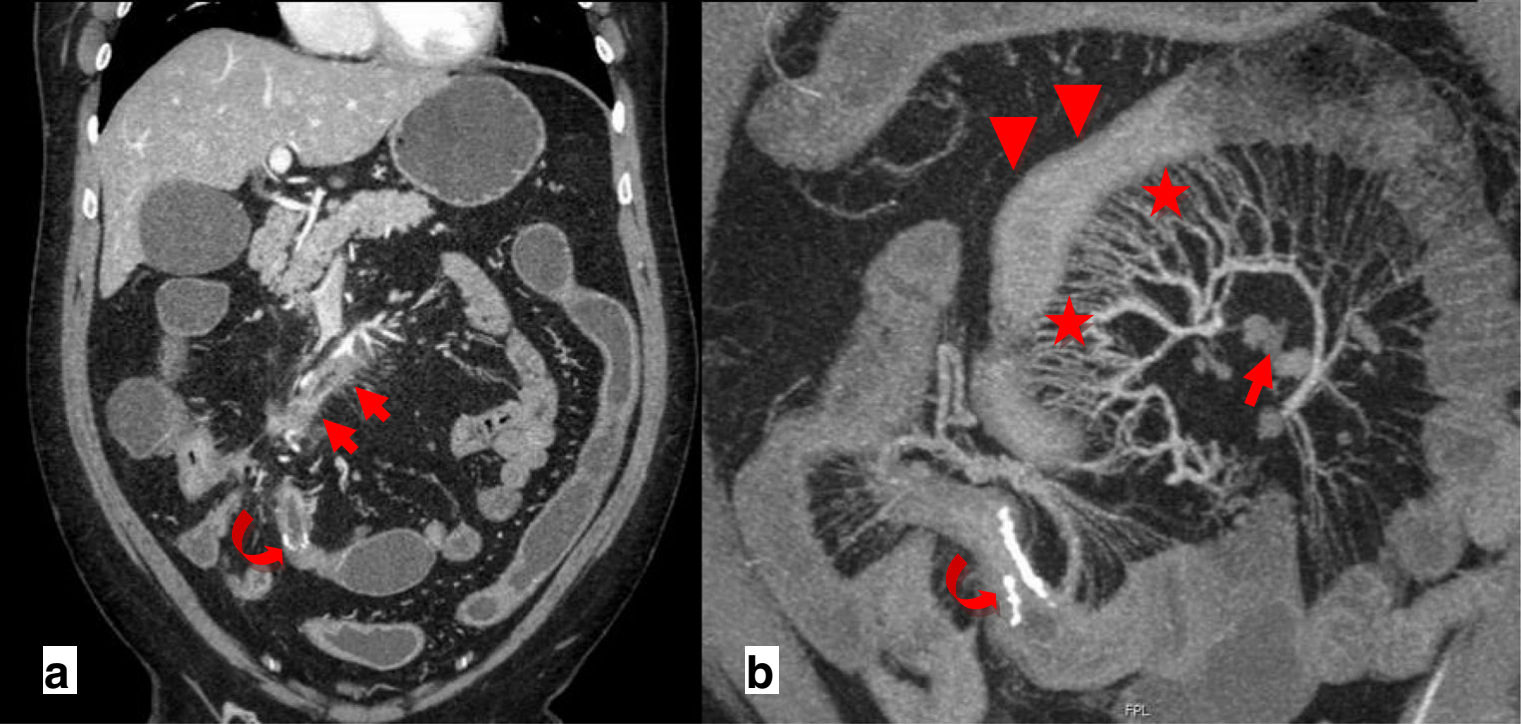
a, b CT enterography of a patient with Crohn’s disease who had previously undergone segmental enterectomy. Note the surgical material at the ileal-ileal anastomosis (*curved arrows in a, b*). There is a fistulous tract between the thickened ileal segment near the anastomosis and the duodenum (*arrows in a*). MIP coronal reformation (*b*) shows mesenteric engorgement (*stars in b*) and lymphadenopathy (*arrow in b*) in the vicinity of a thickened bowel wall segment (*arrowheads in b*)
